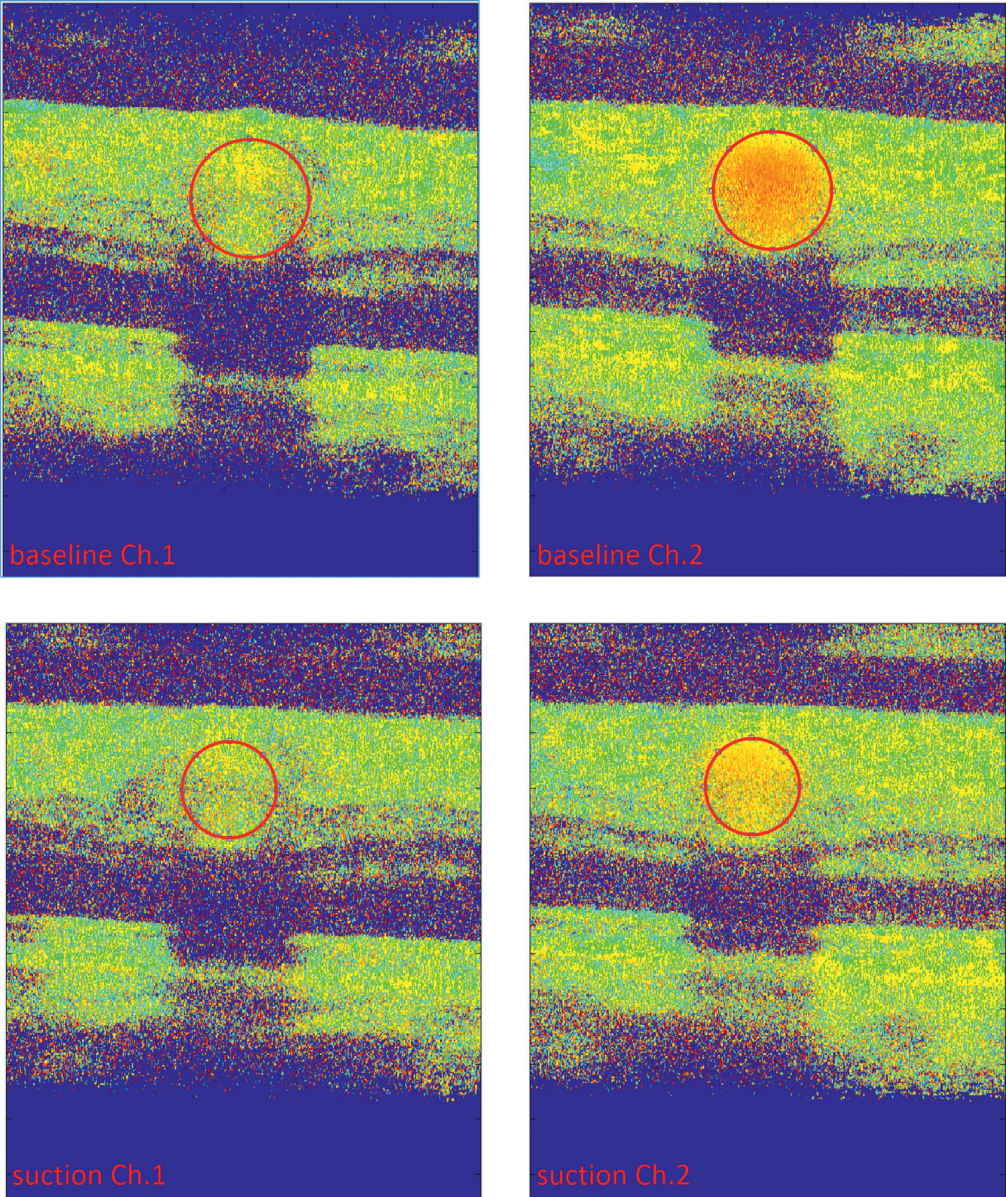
Representative summed phase images of both channels as acquired with the bidirectional Doppler-OCT at baseline (*top*) and during the highest suction level (*bottom*). As for channel 1, the angle of the measurement beam was almost perpendicular to the vessel and the observed phase shift in channel 1 is only small. Vessel diameter was determined based on the channel with the higher phase shift (channel 2). The vessels of interest are marked in *red* and decrease in size upon suction.
